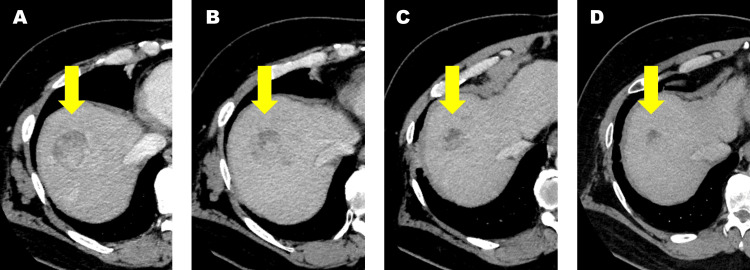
A CT scan of the abdomen. CT images of the lesion located in segment 8 before radiotherapy (A), and at one month (B), three months (C), and six months post-treatment (D). Yellow arrows indicate the targeted lesion.
